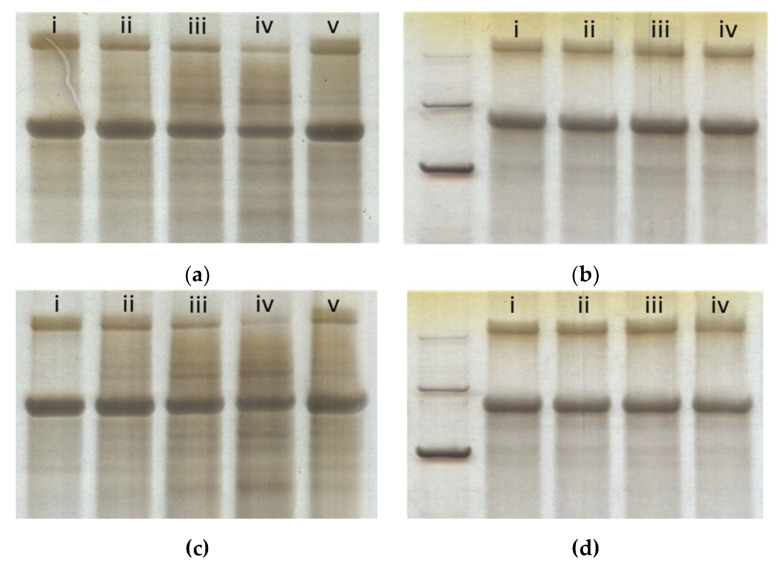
SDS-PAGE gels of ultrasonically atomised RHCIII, (a,c), and non-atomised RHCIII that was exposed to 1.65 MHz ultrasound, (b,d). Samples in gels (c,d) were digested with pepsin, whereas gels (a,b) are their non-digested controls. All lanes marked (i)–(iv) correspond to 0, 20, 60, and 120 min. Lanes marked (v) were re-solubilised printed samples. Molecular weight standards in the left-most lane of (b,d) mark 250, 150, and a high-intensity band at 100 kDa.
